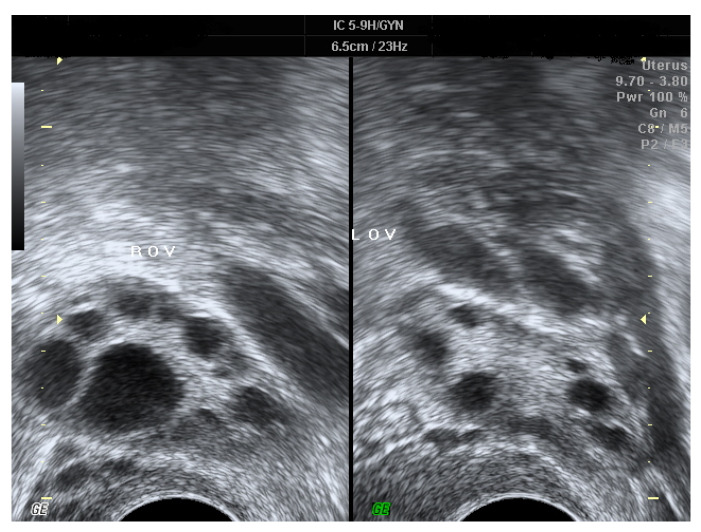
The ultrasonic images of PCOS, which are characterized by the presence of multiple small “necklace-like” follicles in both ovaries and/or increased ovarian volume.
